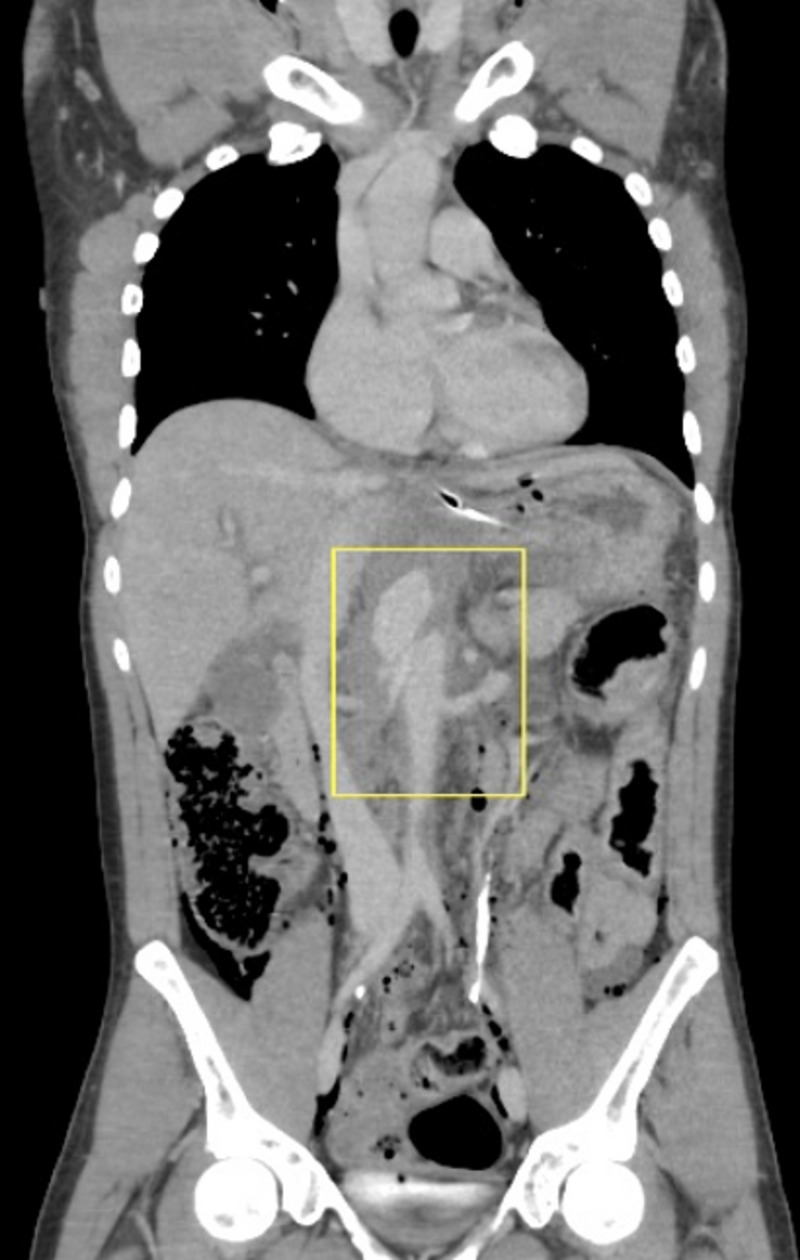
Coronal CT showing aortic dissection at the level of the SMA (box) CT: computed tomography; SMA: superior mesenteric artery
